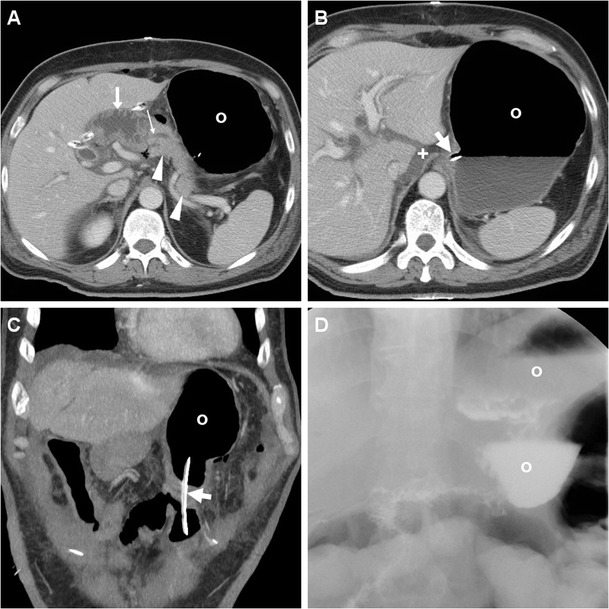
Expected CT findings following uncomplicated PD performed for pT3N0 adenocarcinoma of the Vaterian ampulla. Contrast-enhanced CT (a-c) showed distended stomach (o) with stagnant fluid despite nasogastric intubation (*thick arrows*), consistent with delayed gastric emptying (DGE); normal appearance of the PR (*arrowheads*), the MJL and the PJS with low-attenuation stent (*thin arrow*) in the main pancreatic duct (MPD). In the coronal image (c) the nasogastric tube (*thick arrow*) courses through the GJS. Fluoroscopy (d) confirmed DGE with persistently dilated stomach (o) with stagnant oral CM
